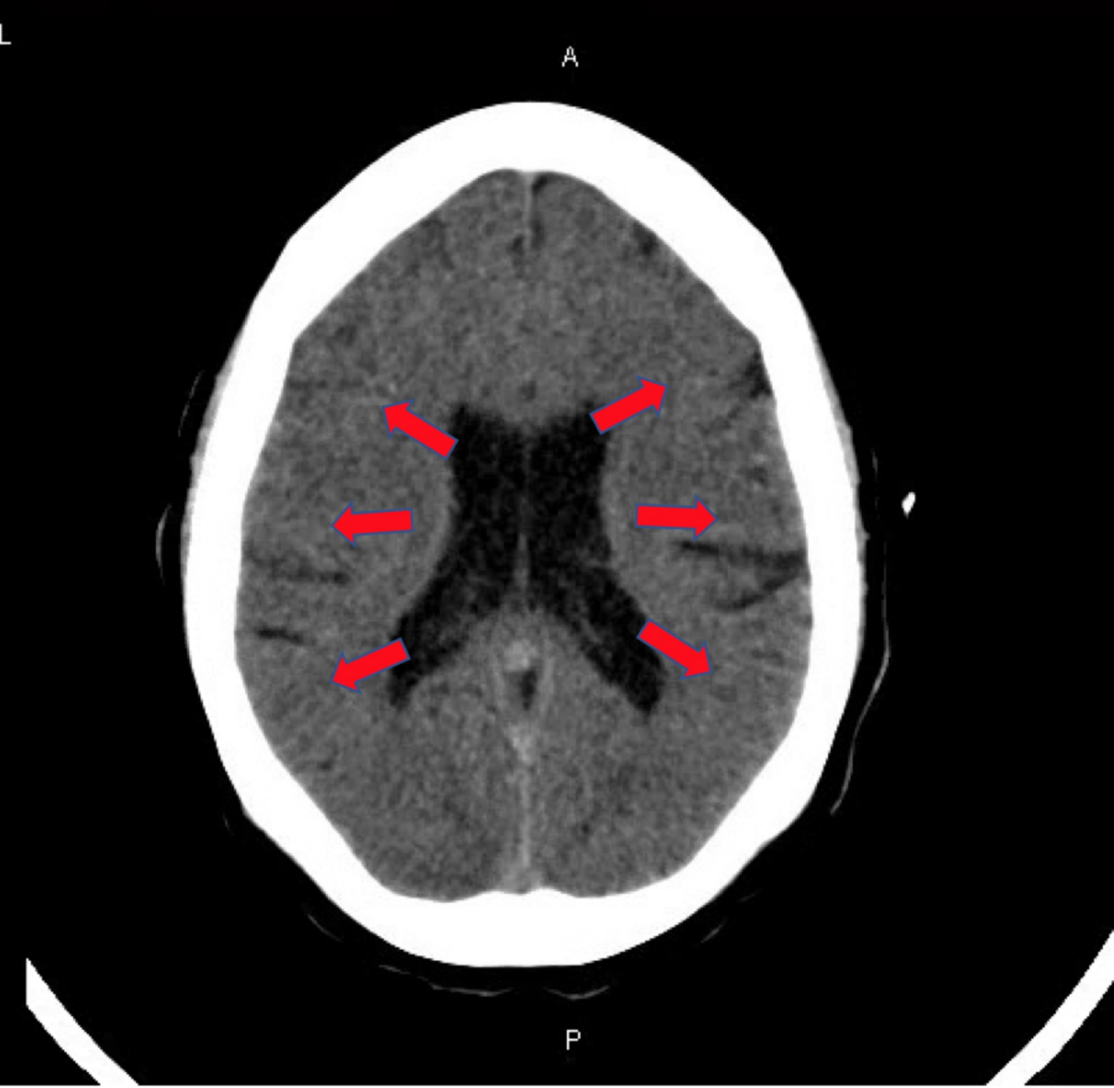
Computed tomography of the head without contrast shows loss of gray-white matter differentiation and diffuse anoxic brain injury consistent with hypoxic ischemic encephalopathy in the setting of cardiac arrest
